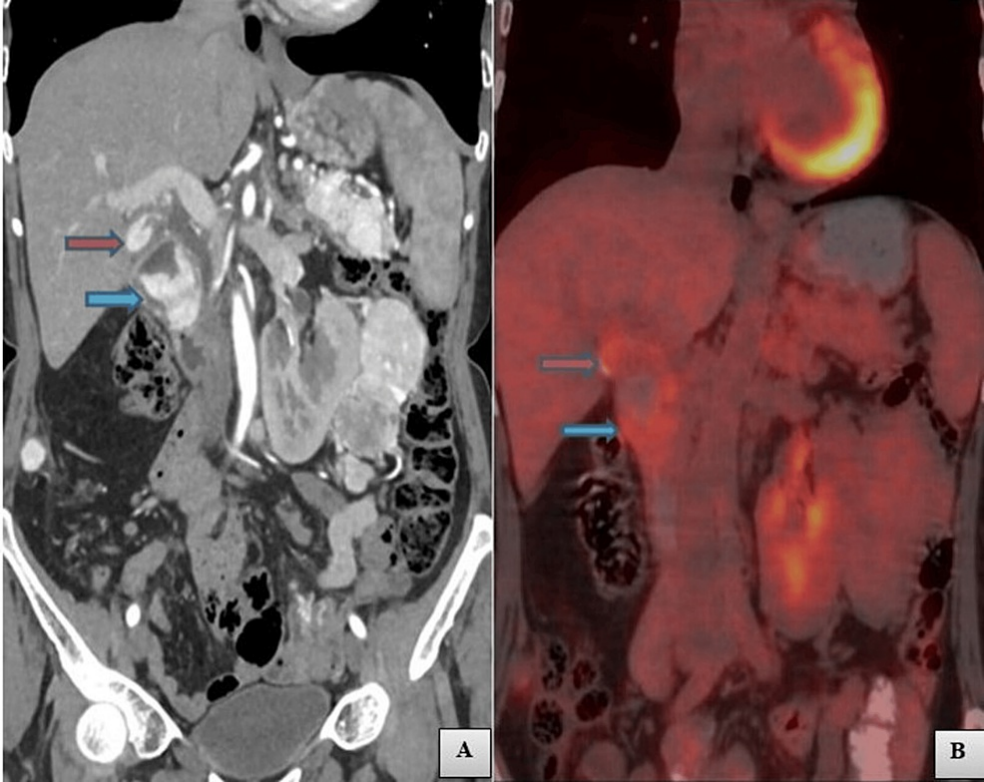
Coronal computed tomography (CT) and positron emission tomography-computed tomography (PET-CT) Figure 4A - Coronal post-contrast CT image showing enhancing lesions in the gallbladder (red arrow) and duodenum (blue arrow). Figure 4B - Coronal PET-CT image showing a significant uptake by the lesion in the gallbladder (red arrow) and duodenum (blue arrow) showing standardized uptake values of 4.5 and 5.1 respectively.
